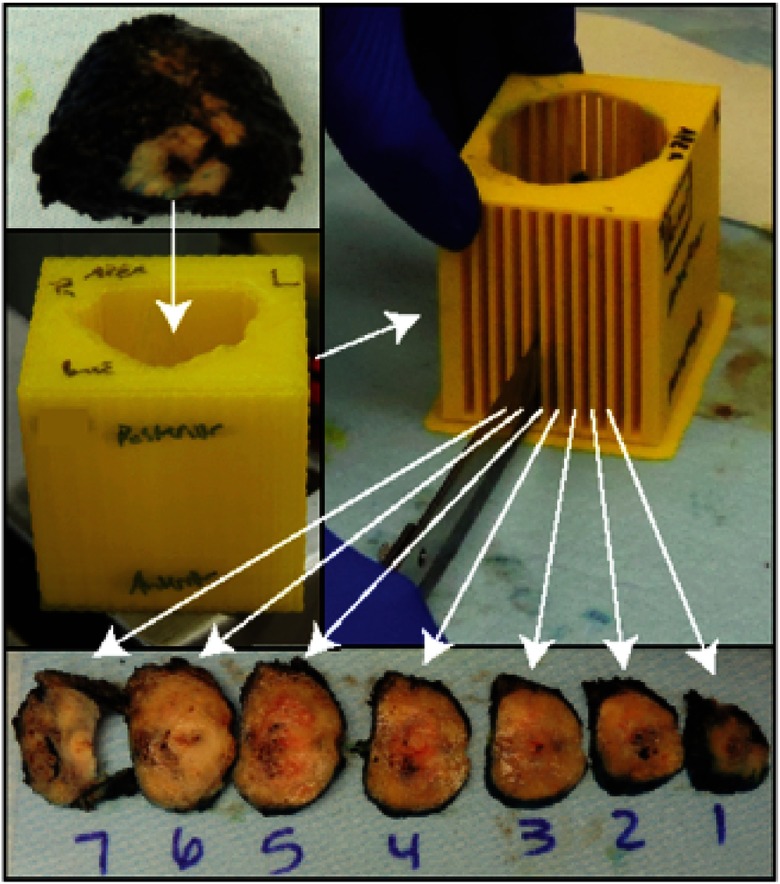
Demonstration of the use of patient-specific 3-D printed prostate slicing jig for sectioning tissue to match the orientation of anatomical presurgical MRI. The posterior surface of the prostate most distorted by the endorectal coil is oriented upward toward the mold insertion opening.
